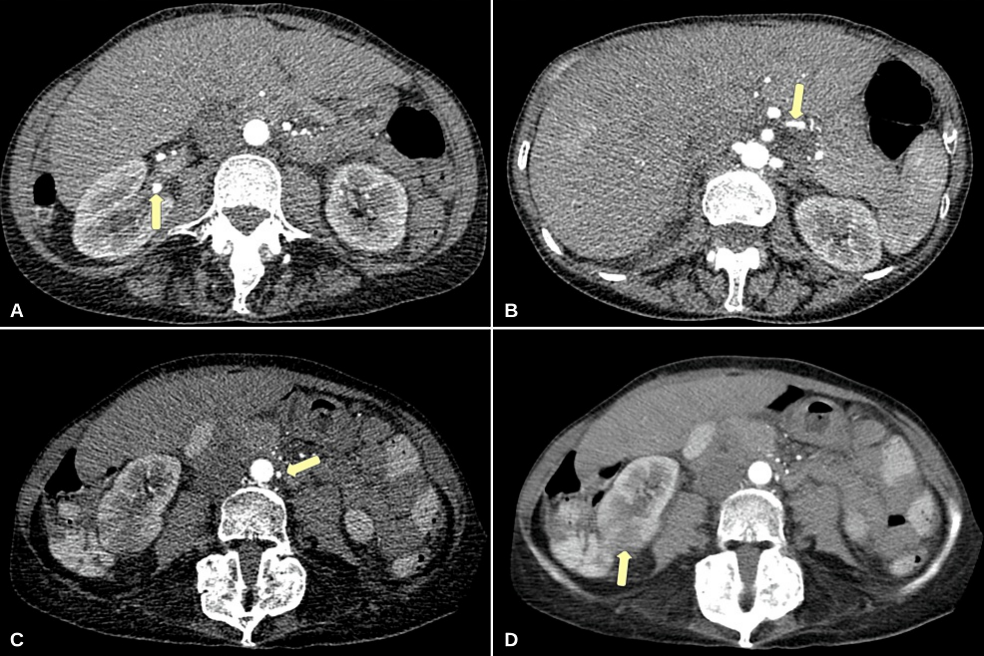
Initial computerized tomography (CT) angiography of the abdomen and pelvis (A) Irregularity of the distal portion of the right renal artery with multifocal aneurysmal dilatation. (B) Slight narrowing at the proximal aspect of the splenic artery. (C) Irregularity and dilatation of the proximal aspect of the inferior mesenteric artery. (D) Heterogenous hypo-enhancement of the posterior aspect of the right kidney.
